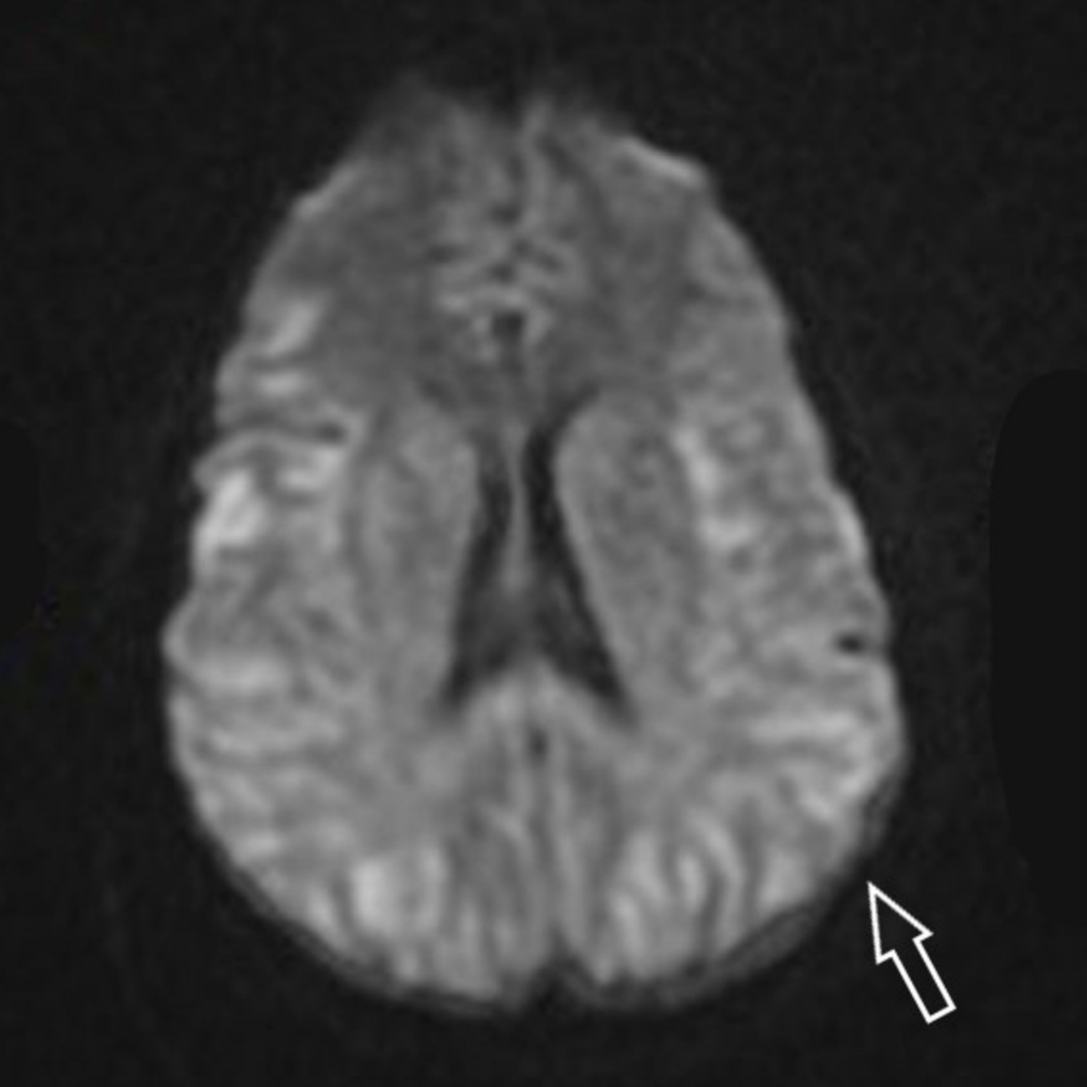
Magnetic Resonance Imaging of the brain showing symmetrically restricted diffusion Arrow over left parietal lobe showing areas of hyperintensity suspicious for hypoxic injury
